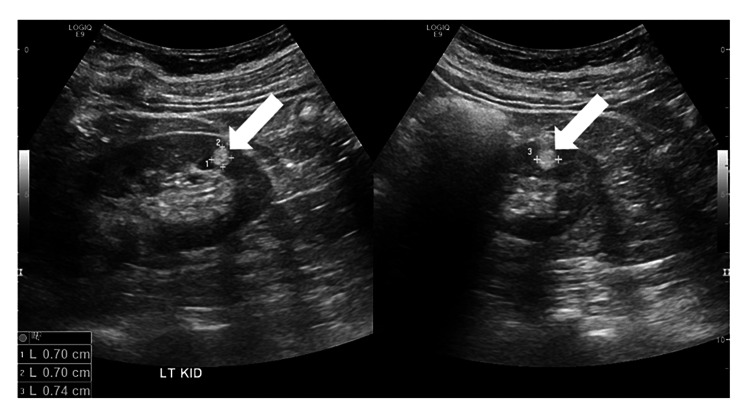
Ultrasound images of the left kidney of a woman with polycythemia rubra vera reveal echogenic lesions interpreted as angiomyolipomas (arrows).
